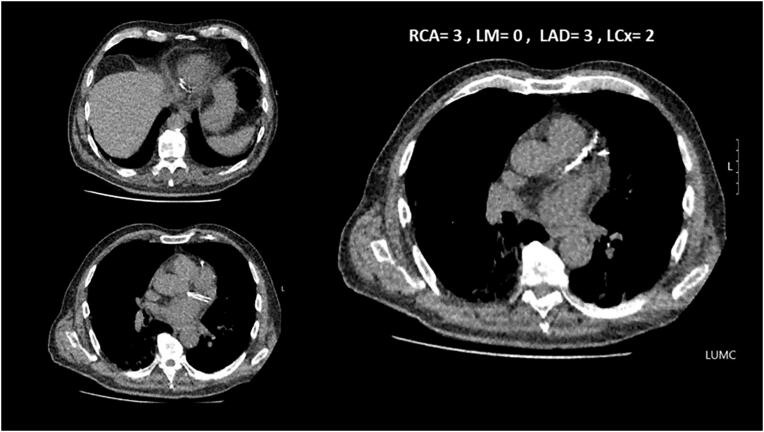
Case example of coronary calcium on chest CT. Example of a patient of 80 years old with a total calcium score of 8, classified as severe [11]. The calcified coronary arteries are the right, the left anterior descending and the circumflex. His medical history reports no previous cardiac problems. During his admission troponin T levels of 17 ng/L were measured, suggesting cardiac injury. Subsequently, echocardiography at 6 weeks post-discharge showed a LVEF of 62%, a LV GLS of −18.7 and a RV strain of −30.0, a TAPSE of 21.9 and S′ of 13. All considered normal [9]. This case represents an example of a patient with clear atherosclerosis and cardiac injury during admission but normal TTE values after 6 weeks.
